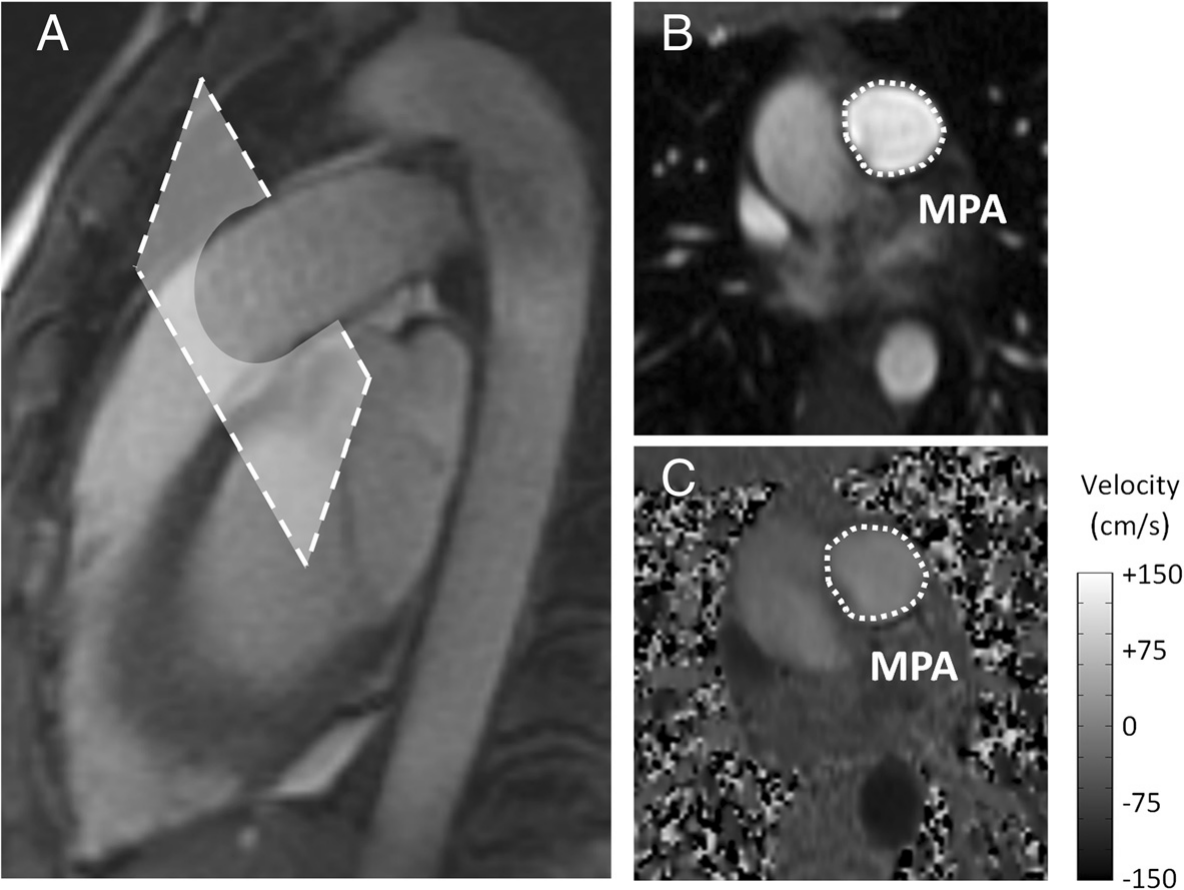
MR images of main PA for a representative subject. a Sagittal image of the MPA displaying the location of 2D-PC acquisition slice. The imaging plane is prescribed in a double-oblique plane perpendicular to the flow direction. b 2D-PC magnitude image of the main PA, used to define and measure the cross-sectional area. c 2D-PC phase image, used to calculate the blood flow velocity, of the MPA. Grayscale-bar represents flow velocity in the phase image (ranges from -150 cm/s to 150 cm/s)
